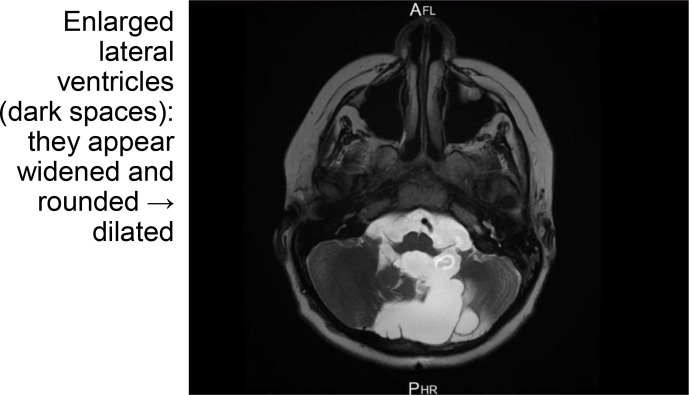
Post-operative axial T1-weighted MRI of the brain following subtotal tumor resection demonstrating persistent supratentorial hydrocephalus. The lateral ventricles appear widened and rounded, consistent with ongoing obstructive hydrocephalus despite surgical intervention. At this timepoint, Visual Assessment 2 confirmed bilateral no light perception with severe optic disc atrophy, prior to radiotherapy initiation. MRI, magnetic resonance imaging.
